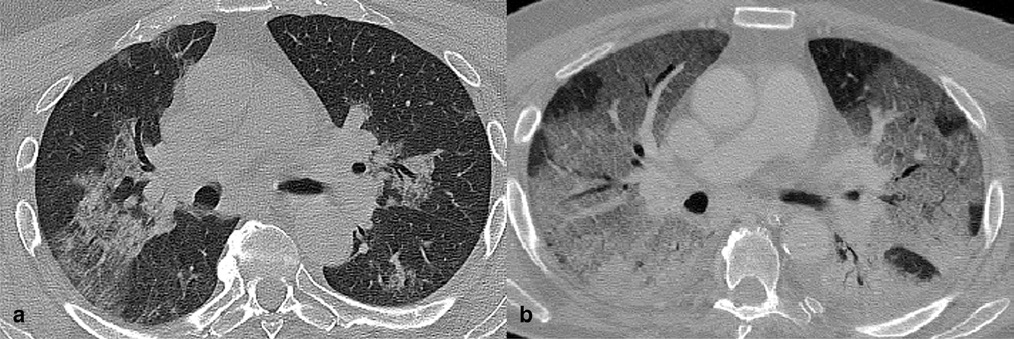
Axial CT image slices of a female COVID-19 patient (α Variant) of 81-year-old. a) CT scan, performed after 1 day from symptoms onset, showed a mixed pattern of GGO and consolidation in upper lobes with peribronchovascular involvement. b) CT obtained after 5 days from symtoms onset. Lesions increased with higher density and superimposed thickening of the interlobular septum in both lungs, with predominant dorsal consolidations.
